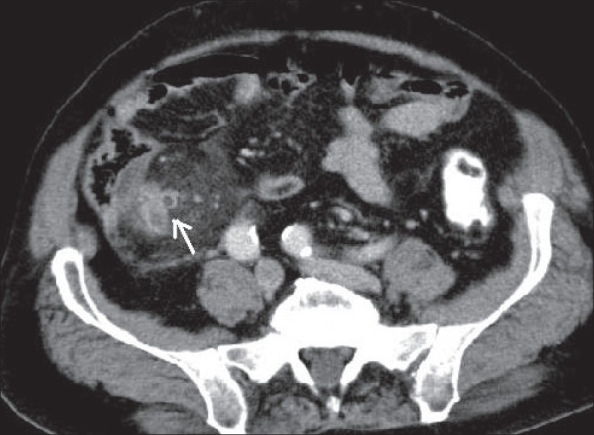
Perforated ileal diverticulitis. Contrast-enhanced CT scan shows multiple ileal diverticuli with an inflamed diverticulum (arrow) in the terminal ileum with adjacent inflammation and a few extraluminal air loculi, consistent with perforation
